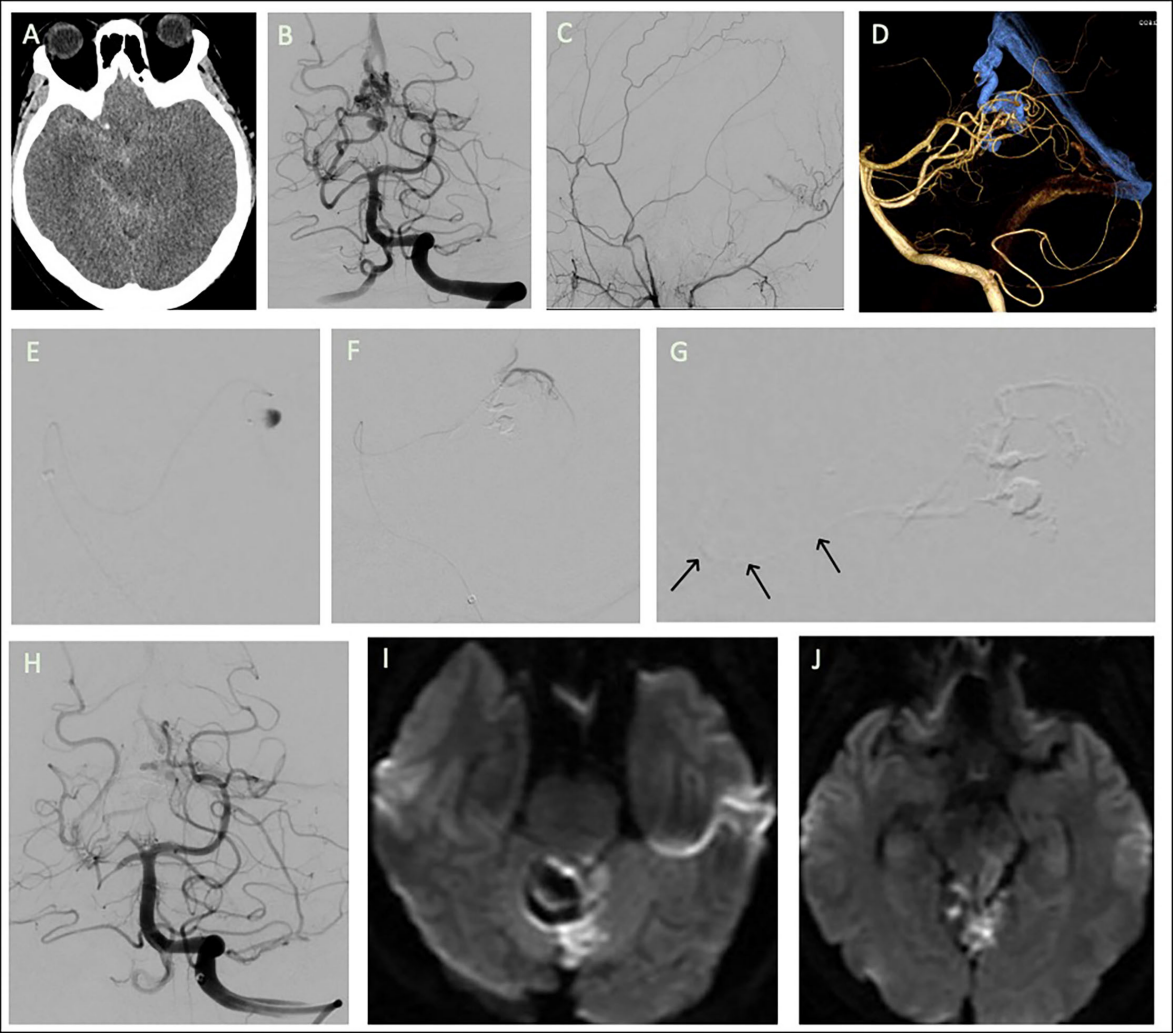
Tentorial dural arteriovenous fistula of case 2. (A) Brain computed tomography revealed diffuse subarachnoid hemorrhage. Angiograms of (B) left vertebral artery (VA) and (C) right external carotid artery. The feeders were the bilateral superior cerebellar arteries (SCAs), bilateral occipital arteries, and left posterior meningeal artery. (D) 3D reconstruction of the fistula. Embolization was performed after navigation of a microcatheter from right SCA to the (E) varix in the draining vein, with most of the fistula being obliterated. The microcatheter then approached the fistula from (F) the left SCA for further embolization; (G) inadvertent flowback of the embolizer to the SCA orifice was noted (arrows). (H) Post-embolization angiogram of left VA injection. (I,J) Follow-up brain MRA revealed acute infarction at tegmentum and tectum of left midbrain and left superior cerebellum.
